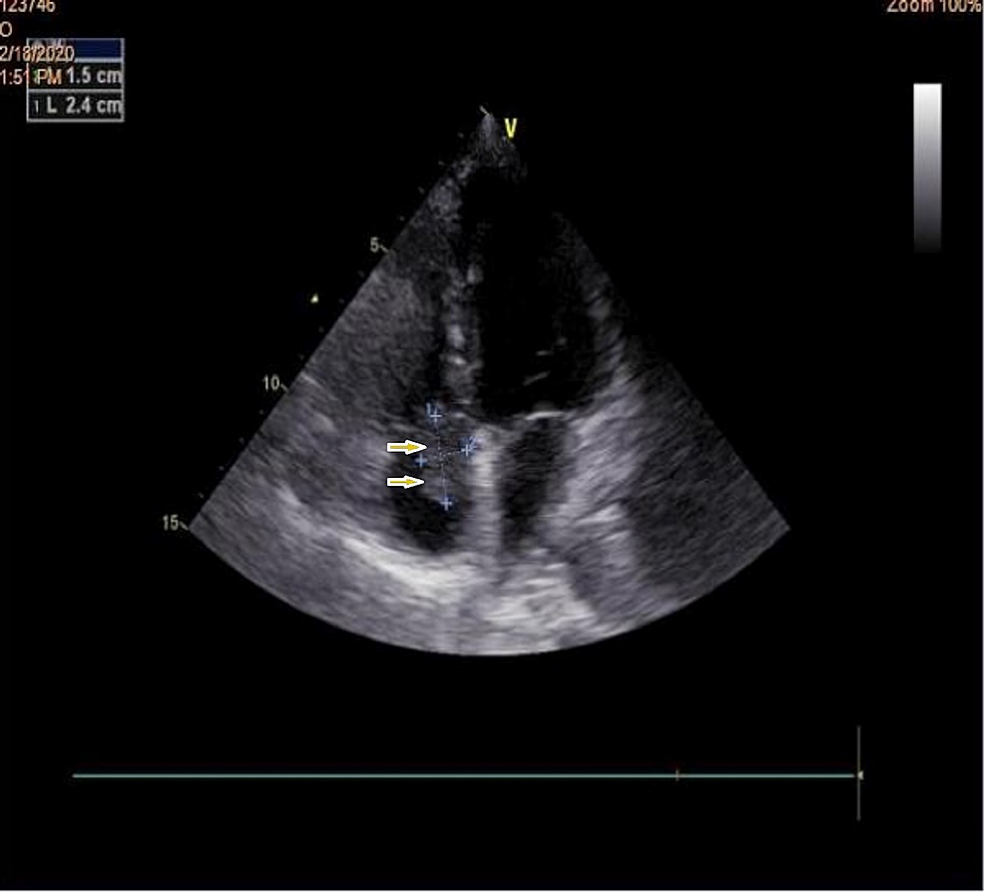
Apical section of four cavities showing the tricuspid mass on echocardiography.
